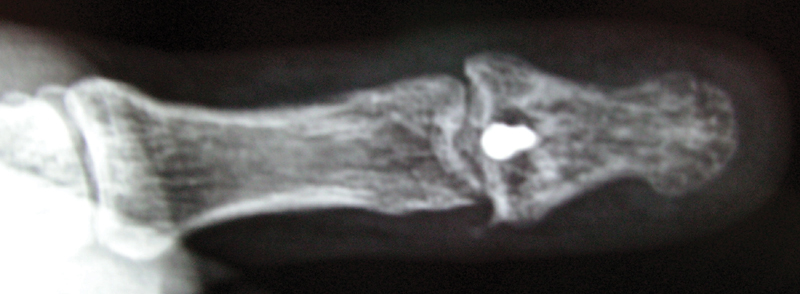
Six months postoperative anteroposterior radiograph.
